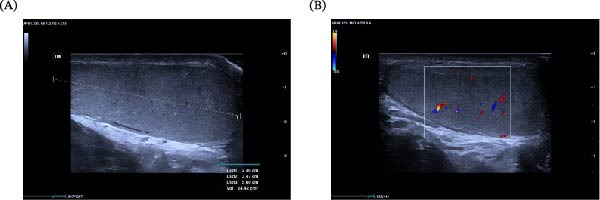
Long‐term follow‐up scrotal ultrasonography and color Doppler imaging. (A) B‐mode ultrasound demonstrating the normal dimensions and homogeneous parenchymal echotexture of the preserved right testis. (B) Color Doppler imaging confirming normal vascularity and robust perfusion within the right testicular parenchyma.
